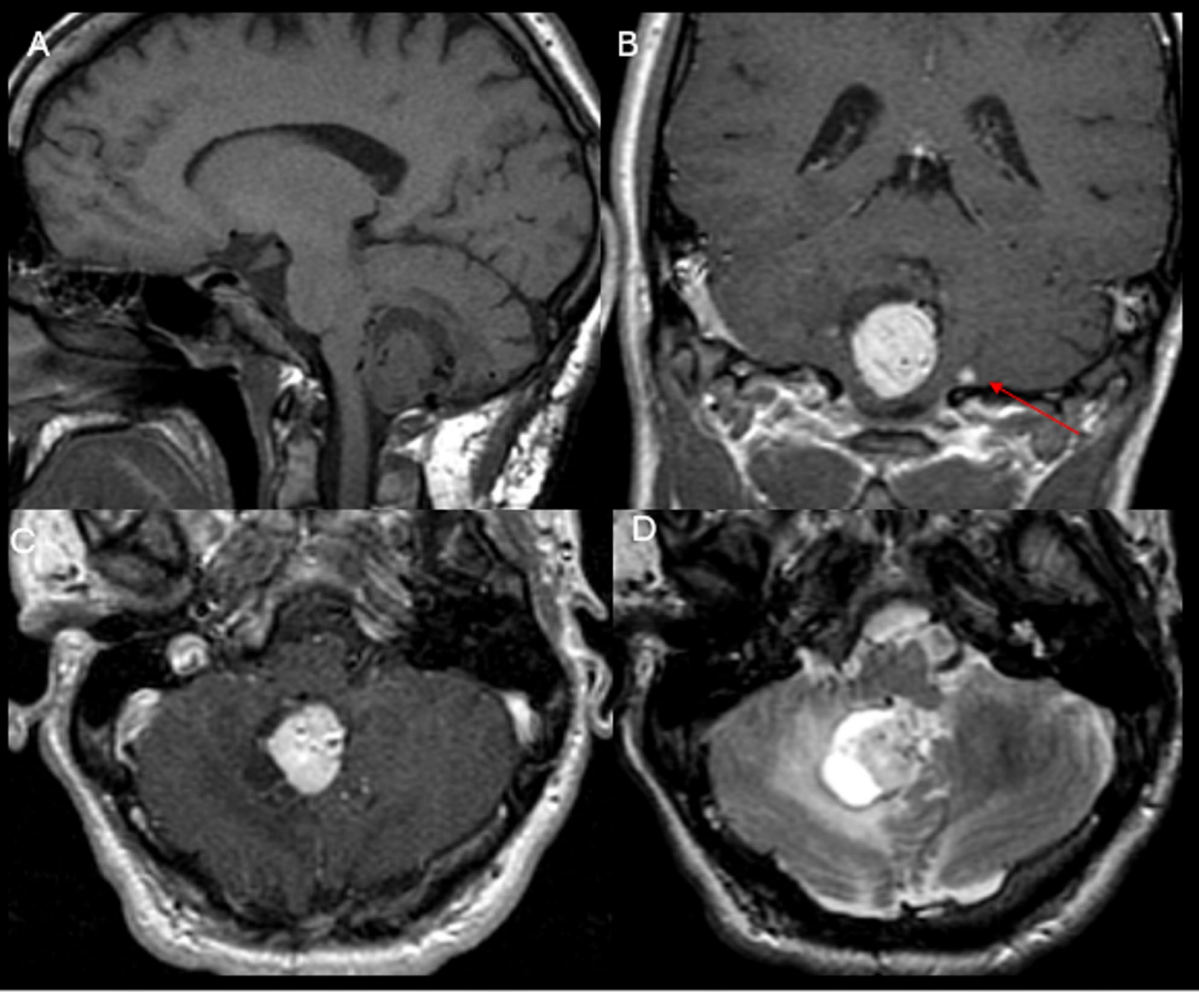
(A) Pre-contrast sagittal T1-weighted image (WI), (B and C) post-contrast coronal and axial T1WI and (D) Axial T2WI. There is a mild enhancing intra-axial mass in the right paramedian inferior cerebellum with associated small cysts and associated perilesional vasogenic edema demonstrating T2 hyperintensity. In addition, a small homogeneously enhancing nodule is present in the inferior aspect of the left cerebellum (red arrow in B). Multiple dilated vessels are noted along the tumor secondary to the characteristic high vascularity of the tumor. These findings are consistent with hemangioblastomas
